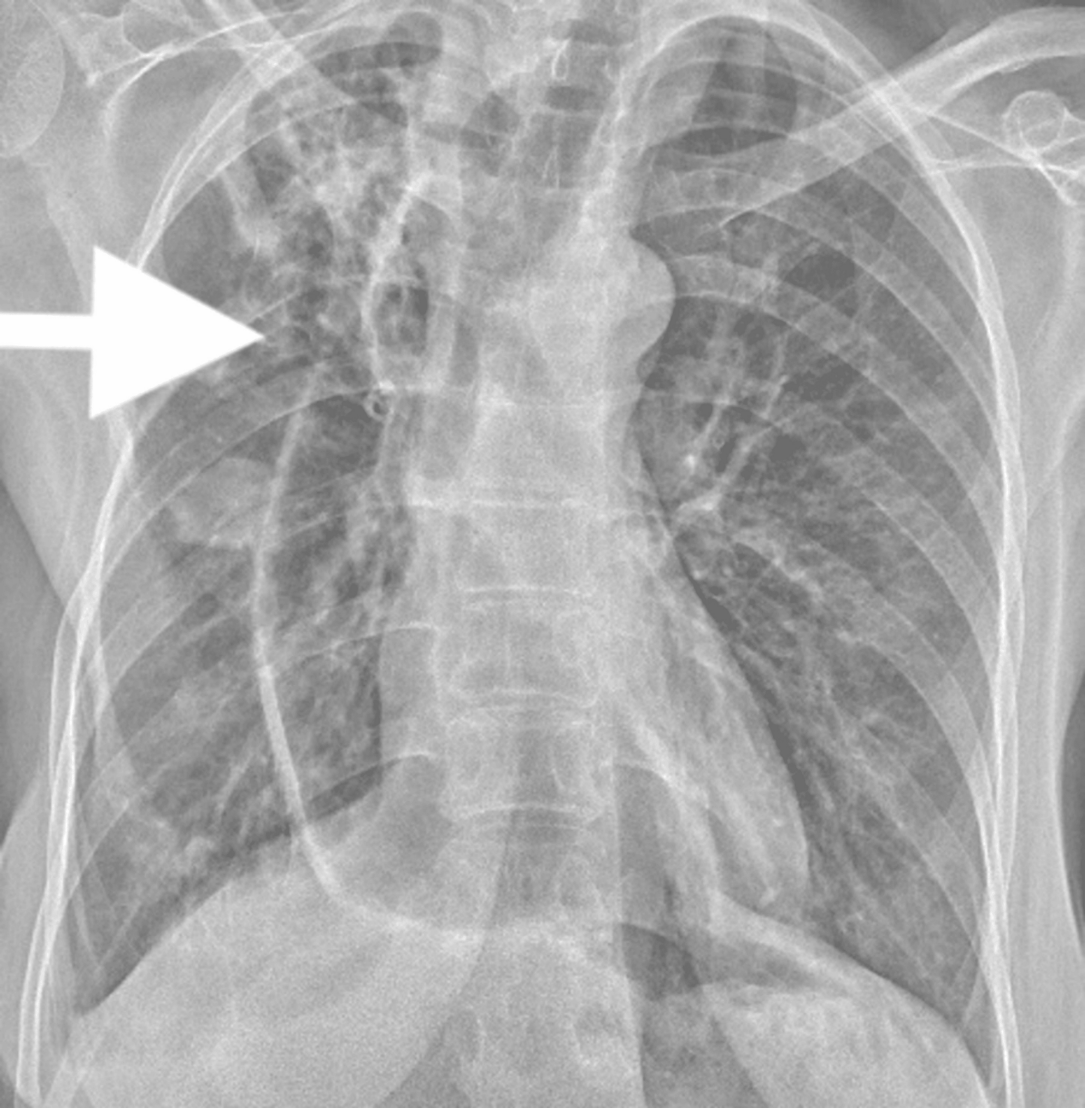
Chest X-ray The arrow indicates a large, lobulated nodularity in the right mid-zone, which is likely indicative of an infection or metastases.
